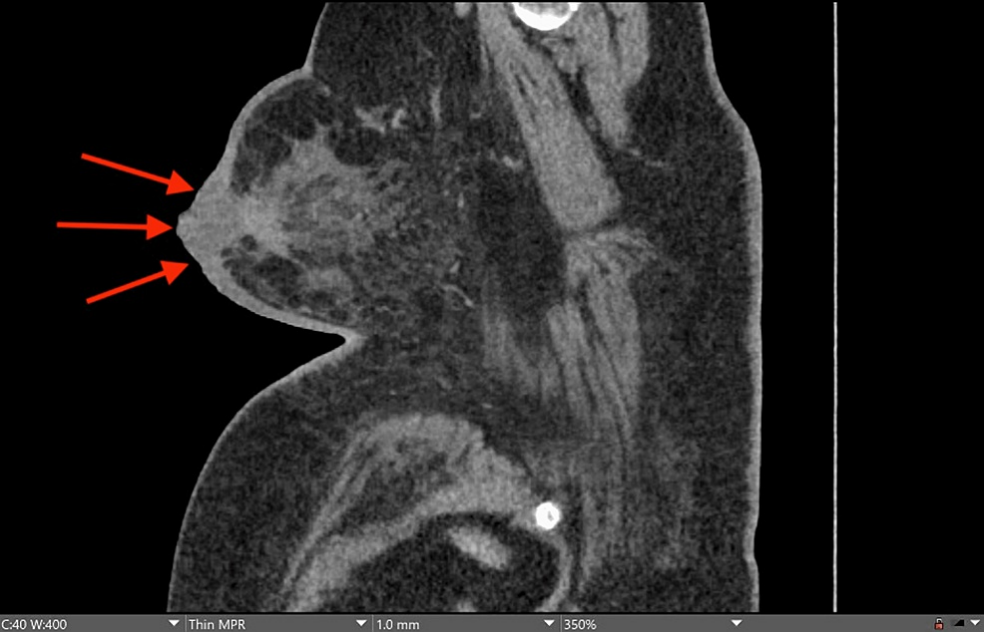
CT scan with a sagittal view. Sagittal contrast-enhanced image demonstrating enhancement and skin thickening of the left breast. The edema and skin thickening are related to prior treatment changes based on the radiologic impression. The red arrows point to the site of the lesion.
